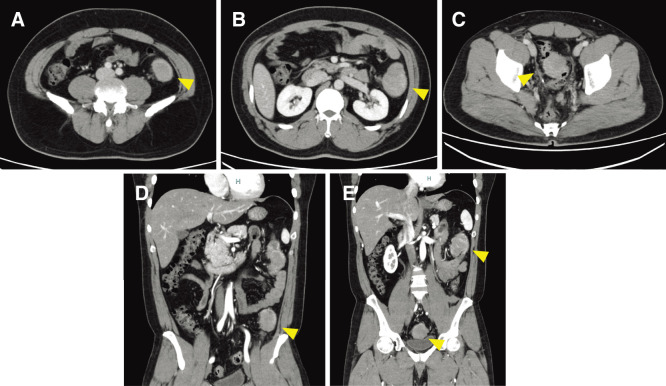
An abdominal contrast CT scan. Three tumors are detected in the descending colon and sigmoid colon. (A–C) Axial views. (D, E) coronal views. Arrowhead: a lead point. (A, D) The tumor was located at the SD Junction. (B–D) The tumors were located in the descending colon and sigmoid colon. CT, computed tomography; SD, Sigmoid-Descending colon
